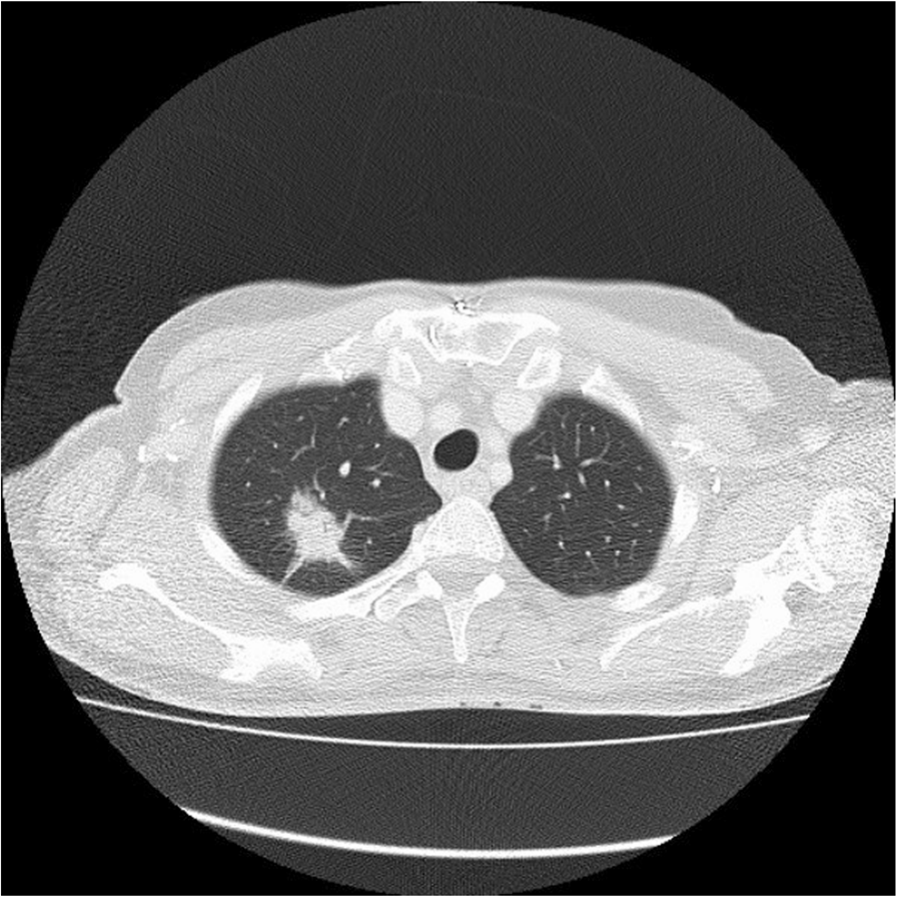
Lepidic predominant adenocarcinoma on CT: 3.2 cm predominantly solid mass in the right upper lobe with air bronchograms, thick spiculations and pleural tethering
